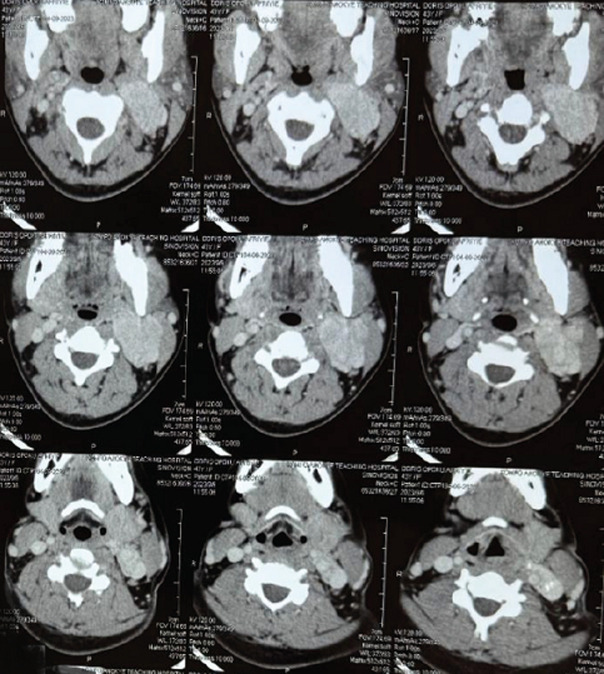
computed tomography angiography of the neck showing the carotid body tumor and its relationship with the internal and external carotid arteries
